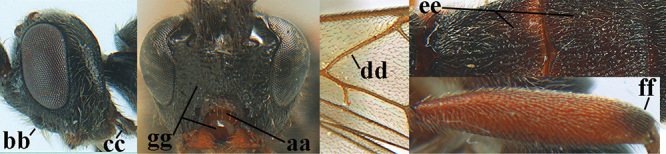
22	Only apical two fifths of marginal cell of hind wing distinctly widened and remainder parallel-sided or nearly so (a), rarely hardly widened apically; vertex flattened behind ocelli (b) and apex of hind femur yellowish or reddish (c); first metasomal tergite with coarse sublongitudinal rugae (d); ovipositor sheath distinctly narrowed apically (e); [vein 1-M of fore wing yellowish brown; basal half of hind tibia pale yellowish/ivory or orange and its apical half black; mesopleuron nearly or completely smooth medio-ventrally; propodeum with pair of crest-like protuberances laterally]	*A. rugulosus* (Nees, 1811)
